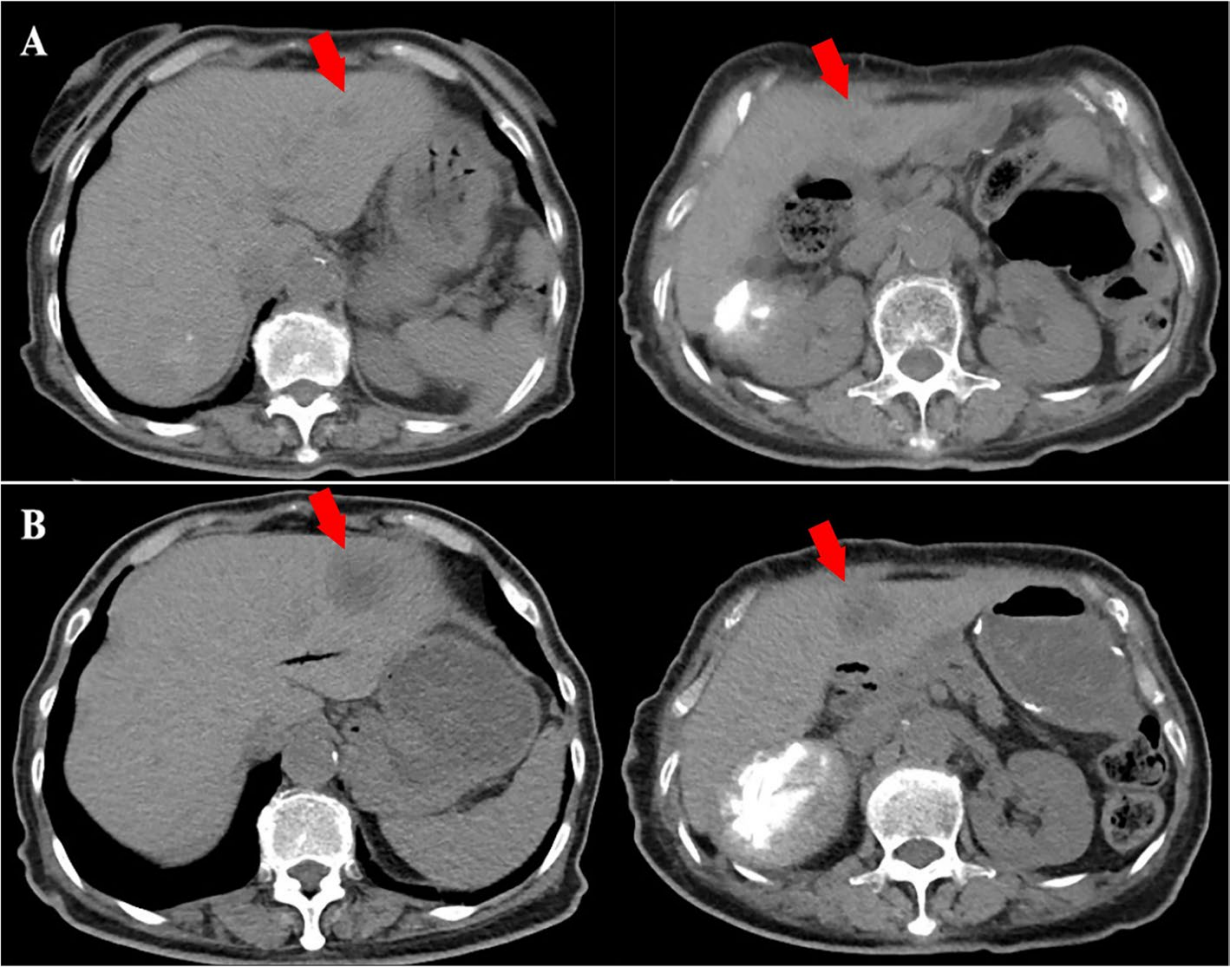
Computed tomography (CT) findings. A Plain CT scan obtained before initiation of treatment with trametinib plus hydroxychloroquine. B Plain CT scan obtained after 8 weeks of treatment shows enlarging hepatic metastatic lesions (arrows)
